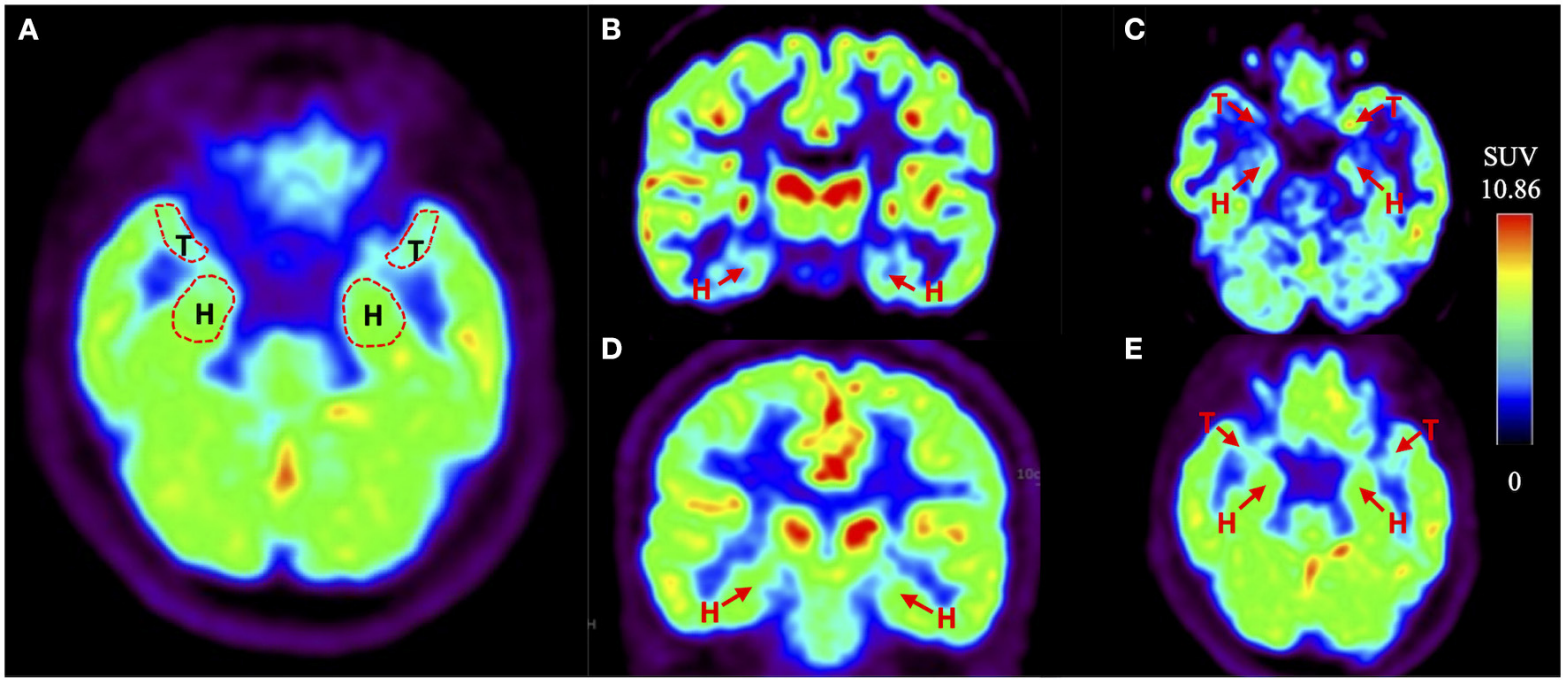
Example of the brain 18F-FDG PET/CT of PD with or without OH. (A) The red dotted line indicates the regions of interest (ROIs), which are bilateral hippocampus (H) and medial temporal lobes (T). (B, C) Shown are coronal and transverse sections of the brain 18F-FDG PET /CT of PD-OH(+) patients. The red arrow points the bilateral hippocampus (H) and medial temporal lobes (T). (D, E) Shown are coronal and transverse sections of the brain 18F-FDG PET/CT of PD-OH(-) patients. The red arrow points the bilateral hippocampus (H) and medial temporal lobes (T).
